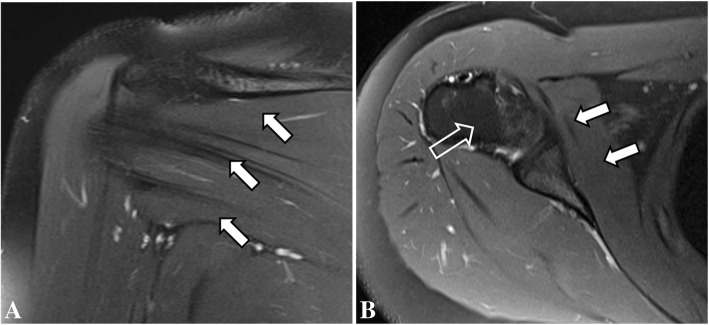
Coronal (a) and axial (b) T2-weighted fat-suppressed follow-up MR images of the right shoulder showing complete resolution of the bone marrow edema of the humerus (open arrow) and of the subscapularis muscle edema (arrows)
